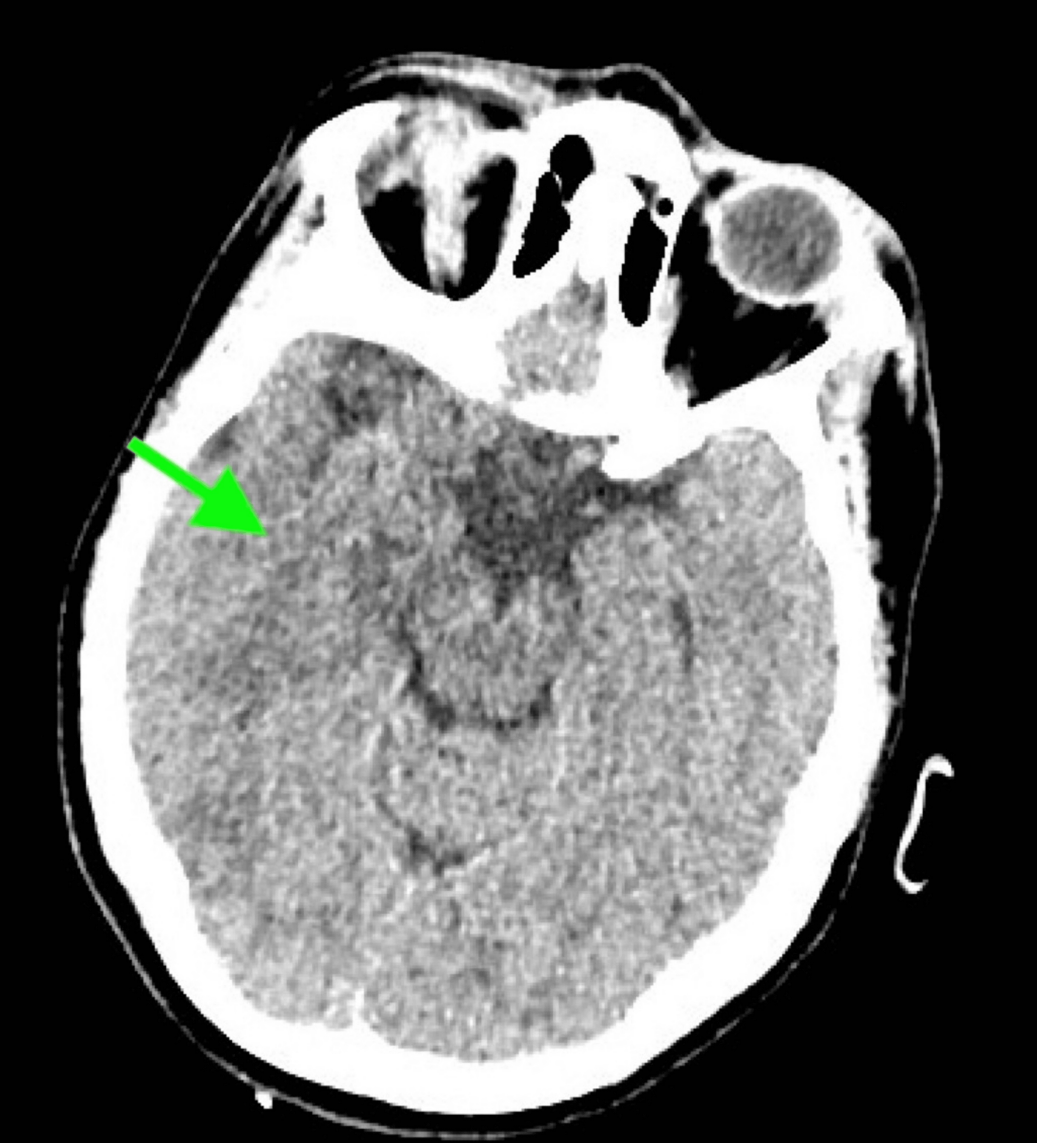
Brain CT showing a hypodense region at the level of posterior limb (posterior crus) of the internal capsule and the lentiform nucleus (green arrow)
